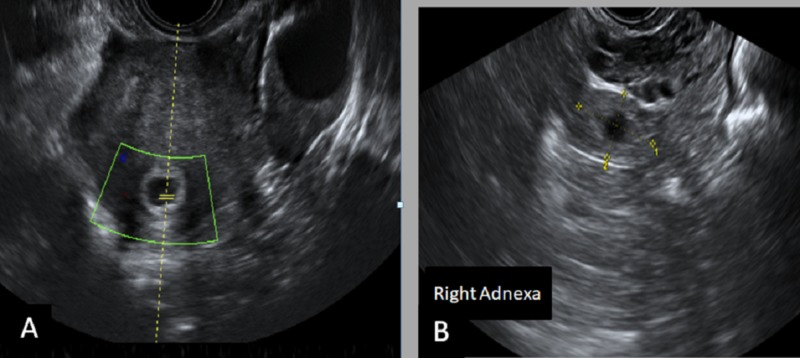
Transvaginal ultrasound A) There is a single nonviable intrauterine gestational sac; B) A rounded echogenic ring-like right adnexal lesion is noted as well. The diagnosis is in favor of heterotopic pregnancy.
